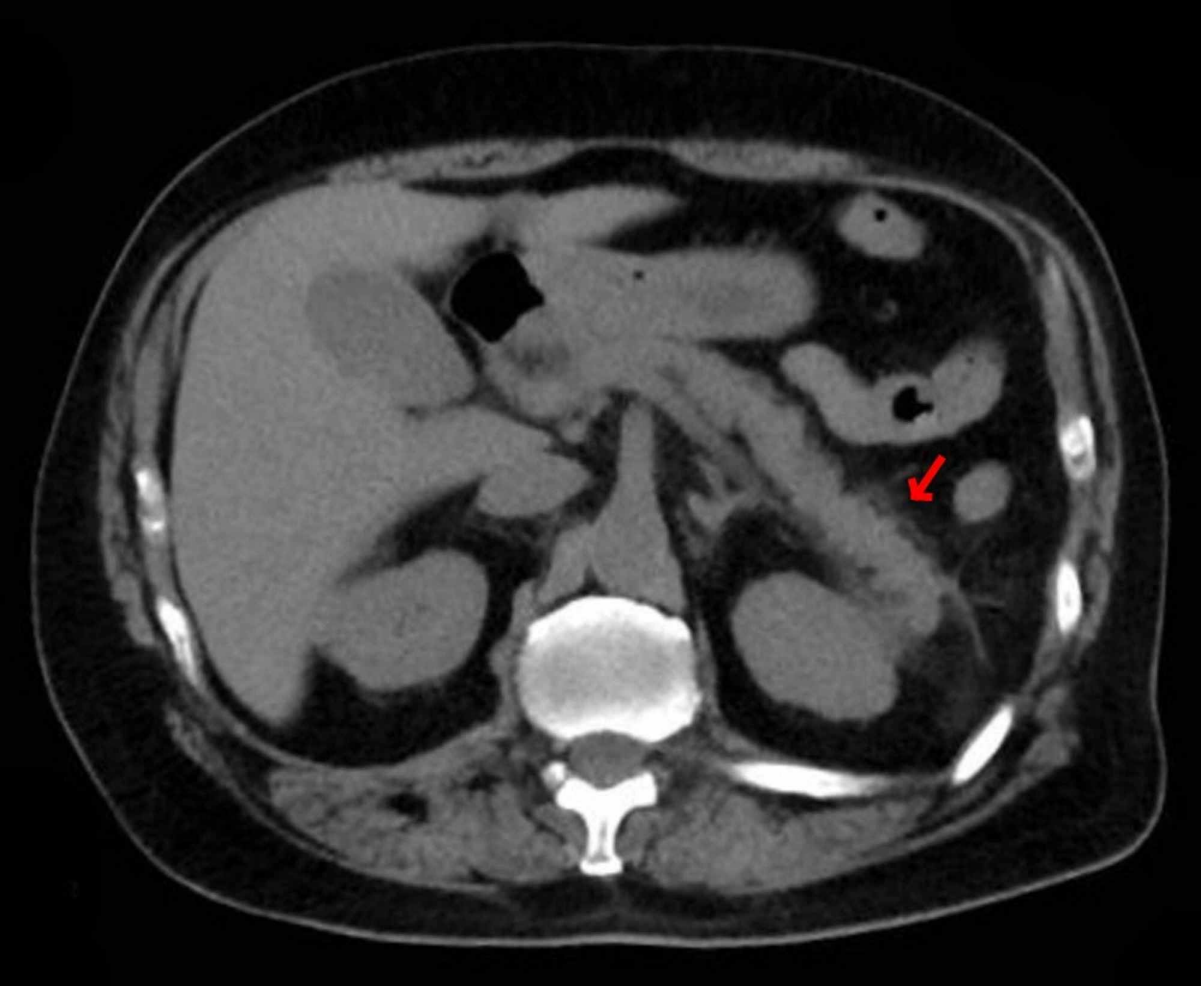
CT abdomen without contrast showing peripancreatic fat stranding greatest around the tail (red arrow). Gallbladder appeared normal without wall thickening or surrounding inflammatory changes and the common bile duct is not dilated.
